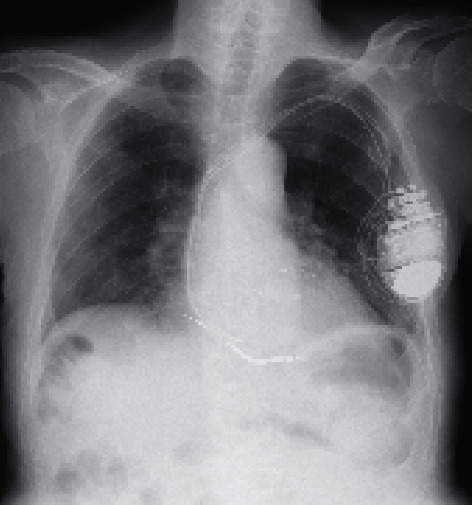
Chest X-ray one day after admission. The free air in the abdomen had disappeared.
